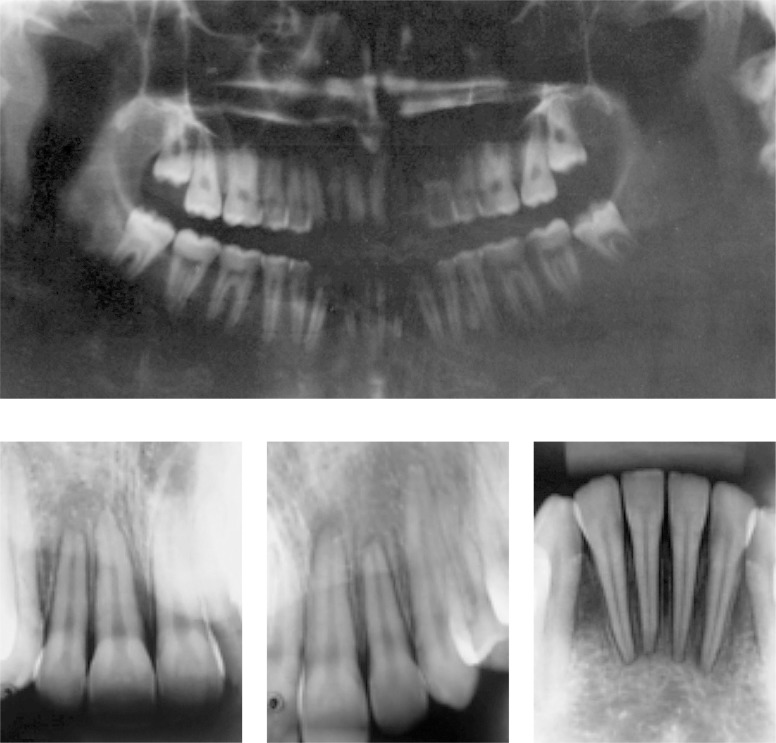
Final panoramic and periapical radiographs of maxillary and mandibular incisors.
